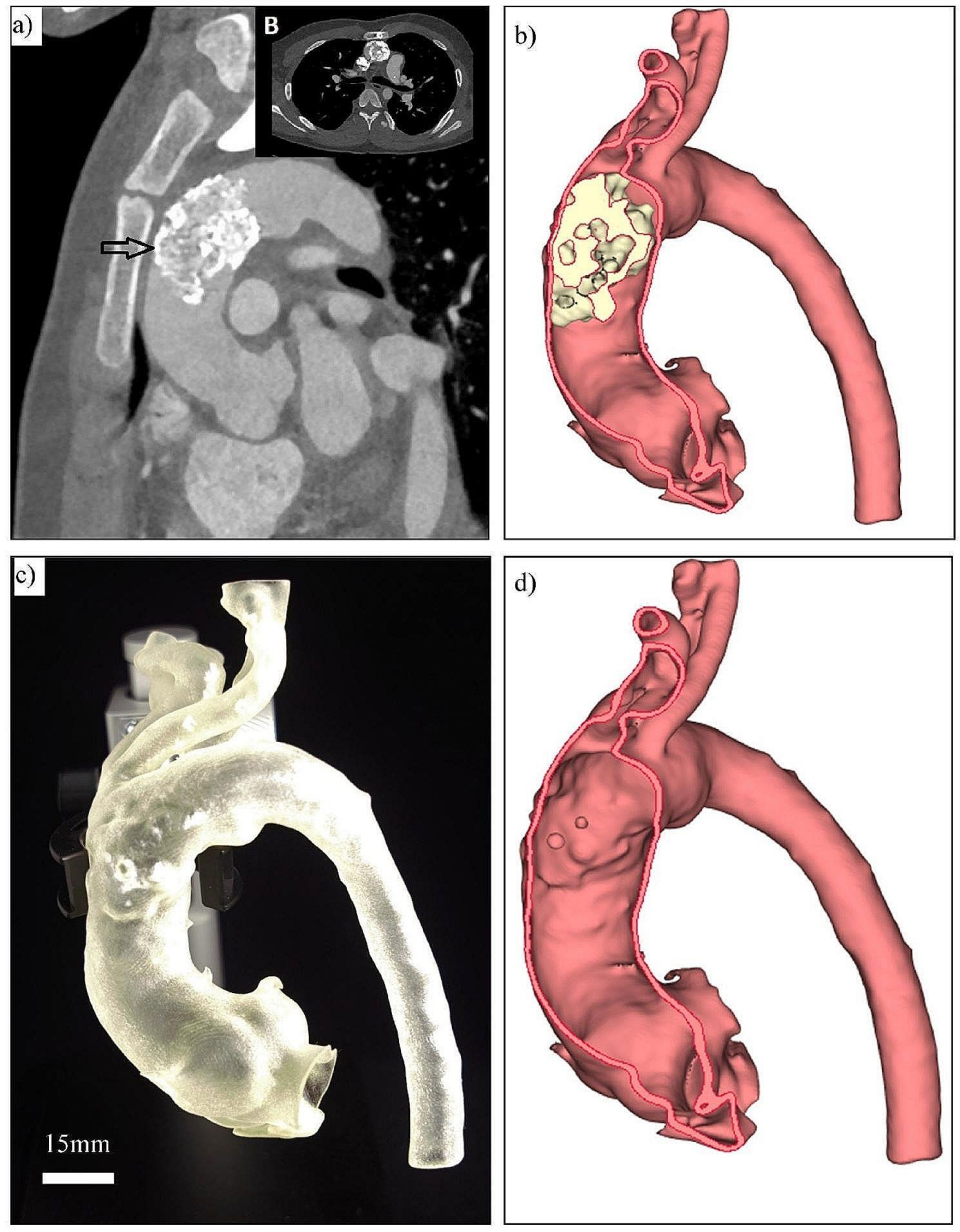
(a) The CT angiocardiography image showing the calcification of the ascending aorta and the arch (arrow) in sagittal and axial views (A and B). Patient-specific reconstruction of the aortic and lesion 3-D model: (b) segmented surface model of the aorta and the calcification region; (c) 3-D printed model of the aorta with vessel mimicking soft texture; and (d) digital surface model of the vessel aorta digital resection
